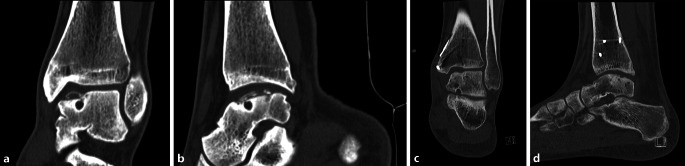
Preoperative and postoperative (1 year follow-up) computed tomography (CT) scans of the same patient. a Coronal preoperative CT scan. b Sagittal preoperative CT scan. c Coronal postoperative CT scan. d Sagittal postoperative CT scan
